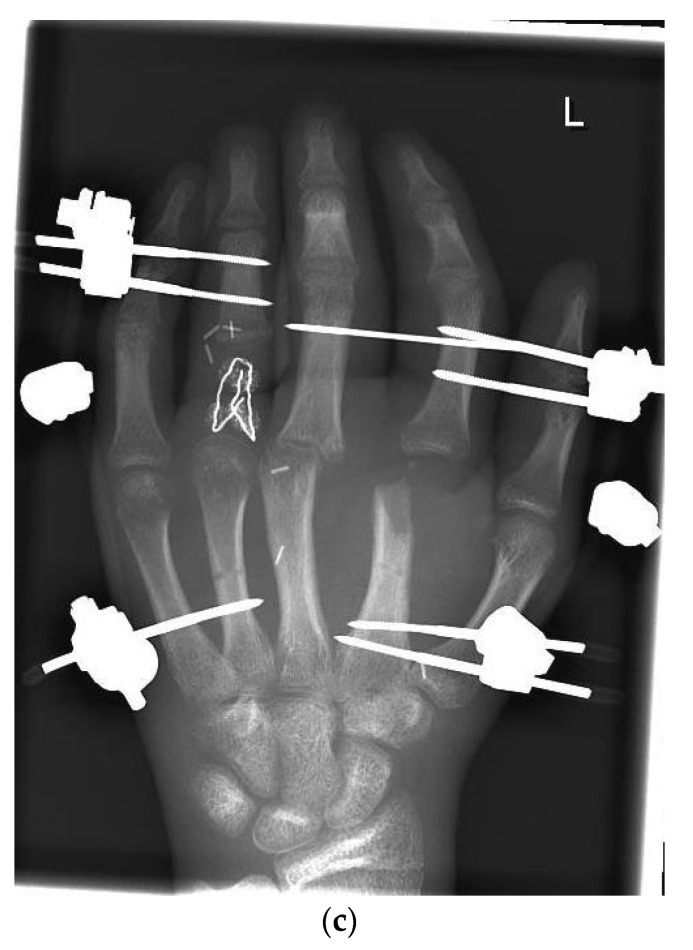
(a) Preoperative wound with exposed bone defect at MCP II–IV. (b). Preoperative X-ray with the destruction of MCP II–IV. (c) X-ray after the first surgical treatment using external fixators.
